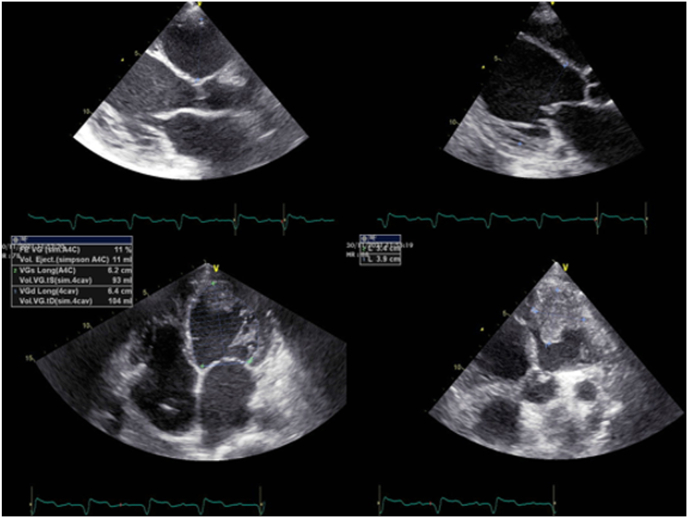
Echocardiogram showing biventricular enlargement with depressed left ventricular ejection fraction and a large apical thrombus.
